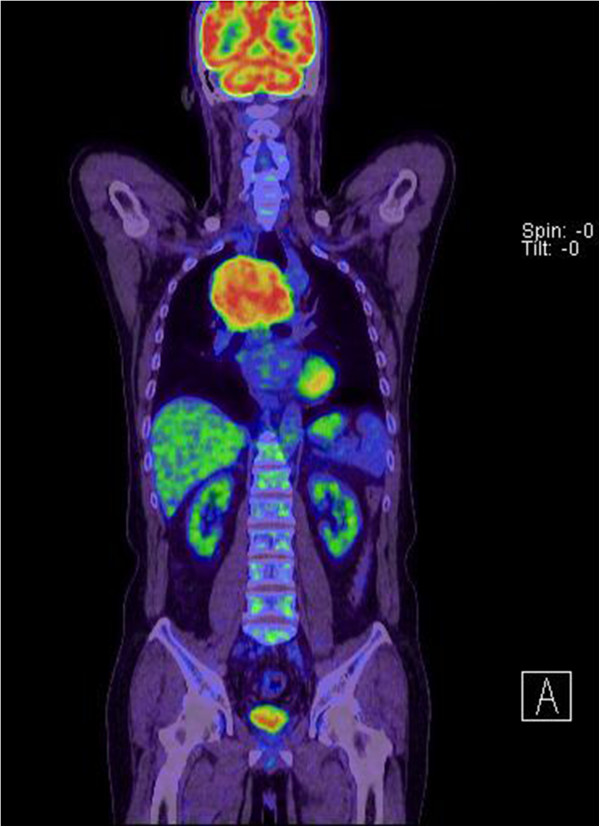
Hypermetabolic activity (SUVmax: 9.9) was shown by positron emission tomography.
